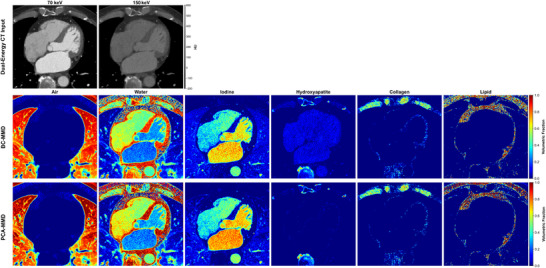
Representative patient results showing six‐material decomposition (air, water, iodine, hydroxyapatite, collagen, and lipid) obtained from dual‐energy CT images reconstructed at 70 and 150 keV. Top row: Input virtual monoenergetic images (WW/WL = 400/50 HU). Middle row: Baseline BC‐MMD decomposition results. Bottom row: Proposed PCA‐MMD decomposition results. Compared with the baseline, PCA‐MMD demonstrates improved confinement of the iodine signal to contrast‐enhanced heart substructures and reduced cross‐talk into noniodine materials, yielding more spatially coherent and anatomically plausible material fraction maps.
